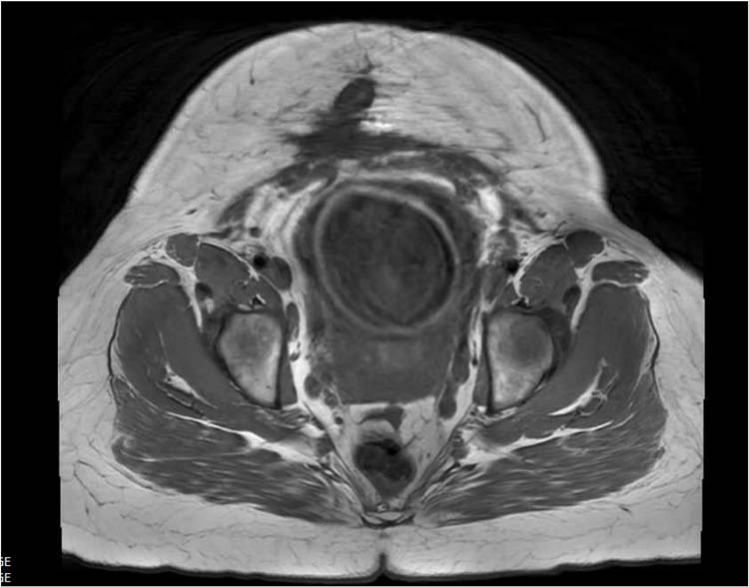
Abdominal MRI scan showing ventral wall defect.
